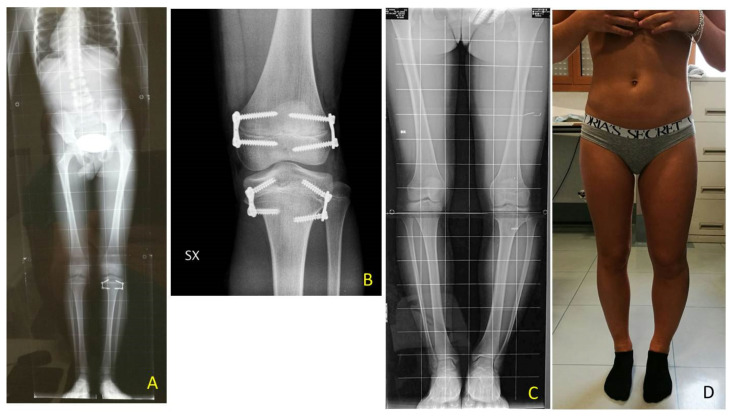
BWS (Beckwith-Wiedemann syndrome) female patient with severe LLD (leg length discrepancy) left. At age of 7 years with a length discrepancy of femur of 1.5 cm and of 3.5 cm of tibia a PTE (temporary proximal tibia epiphysiodesis) was performed: (A) at age of 9.4 years femur discrepancy of 3.0 cm was present leading to a severe pelvis obliquity without shoe lift correction as shown in X-ray od spine and lower extremities; (B) postoperative X-ray in antero-posterior projection showing performed DFE (temporary distal femur epiphysiodesis); (C) at age of 12.7 years, after reaching leg length equality, removal of implants was performed. X-ray showing screw breakage on lateral aspect of tibia; (D) clinical aspect at follow-up with mild varus knee deformity bilaterally and nonpelvic obliquity.
